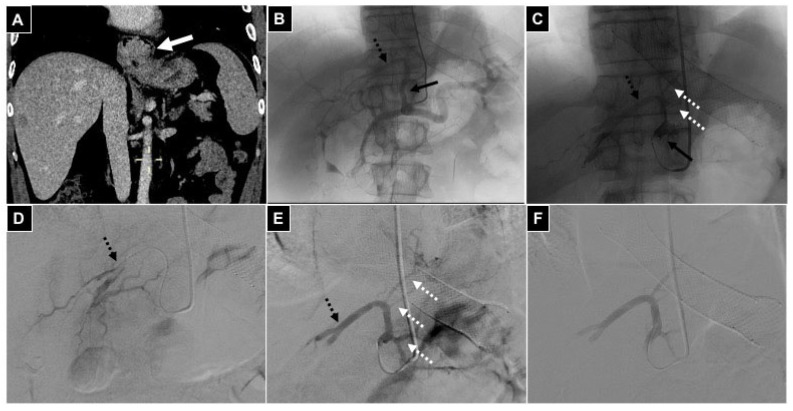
A 42-year-old male with gastric adenocarcinoma involving the cardias region and causing chronic anemia; palliative bland embolization is selected. (A) Coronal CT scan in venous phase showing the neoplastic lesion (white arrow), and a liver metastasis is also evident in segment VII. (B) Selective coeliac trunk arteriography is acquired via a 5 Fr diagnostic catheter positioned via radial access, and gastrohepatic trunk variation is evident (black arrow), with the left hepatic artery (black dotted arrow) originating from the left gastric artery; a palliative gastrooesophageal metallic stent is appreciable too. (C) A 2.4 Fr microcatheter is positioned into the gastrohepatic trunk (black arrow) and DSA is acquired showing small branches refurnishing the tumor (white dotted arrows) not suitable for microcatheterism, and the left hepatic artery (black dotted arrow) is evident. (D) The microcatheter is advanced into the left hepatic artery (black dotted arrow). (E) After injection of Embocept-S^®^ microparticles, temporary embolization of the left hepatic artery (black dotted arrow) is obtained and arterial flow is diverted to the lesion (white dotted arrows), so embolization with definitive 500–700 μm microparticles is conducted. (F) Final DSA control showing no more opacification of left gastric branches and left hepatic artery.
